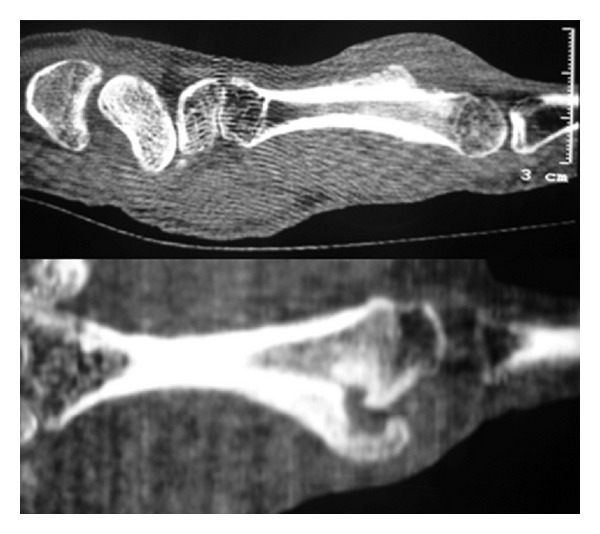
CT sagittal images of the second metacarpal demonstrating saucerization of the underlying cortical bone.
